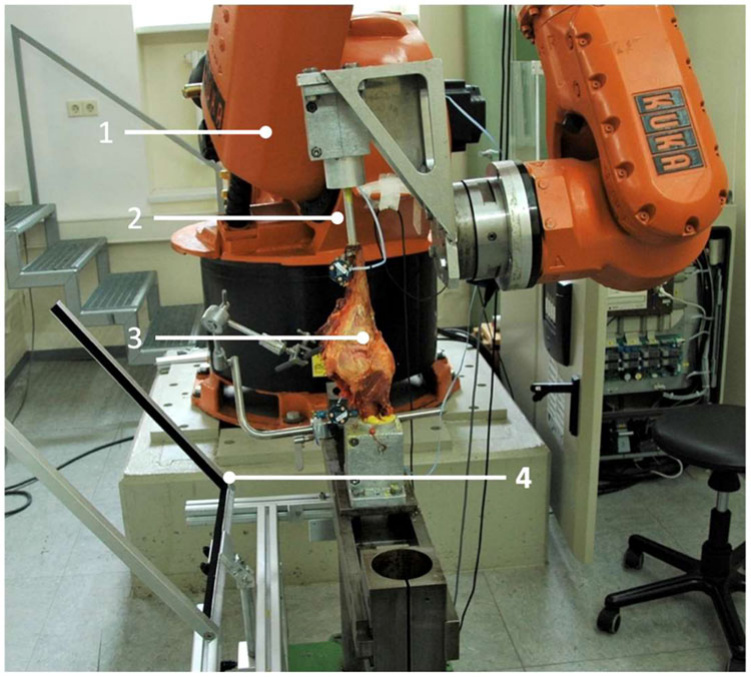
Left knee (3) mounted upside‐down in the robotic test setup (1). To avoid obstruction with the moving arm, the knee was extended using a tibial nail (2). After applying valgus stress, an anterior‐posterior radiograph could be performed using a detector screen, which was mounted in a custom‐made fixture (4). The construct could be angulated to account for knee alignment in the robot and flexion angle.
